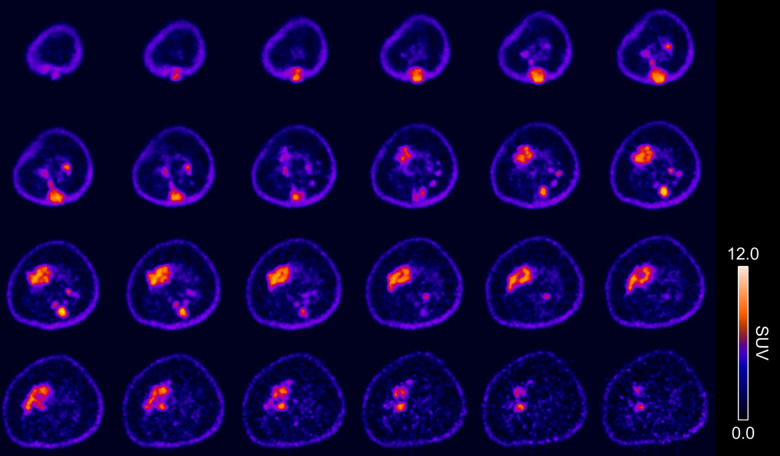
Prone-position [^18^F]FDG PET image of patient with recurrent intraductal carcinoma of right breast. High spatial resolution and target-to-background contrast enable clear lesion delineation and detailed visualization of tumor heterogeneity.
